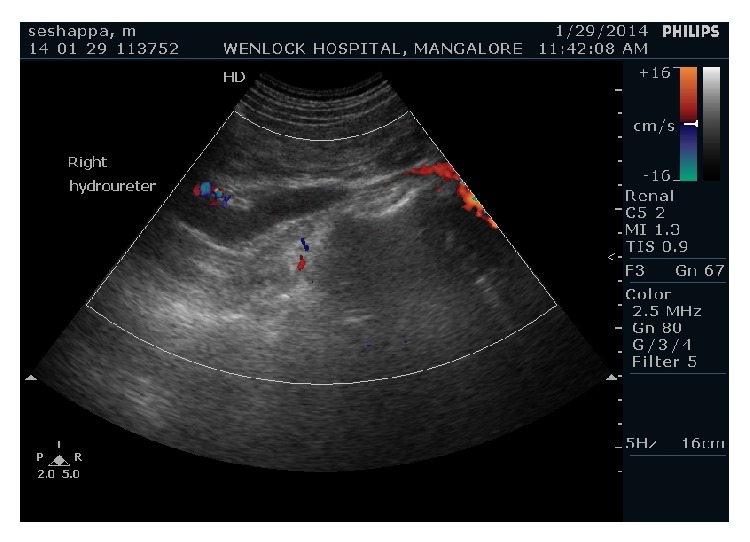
Ultrasound examination with Doppler shows abrupt narrowing of the dilated right ureter at the level of right iliac vessels.
